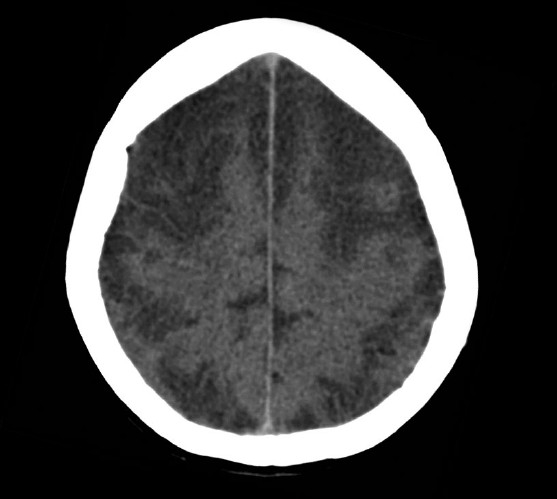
CT brain with contrast, day 5 in ICU
